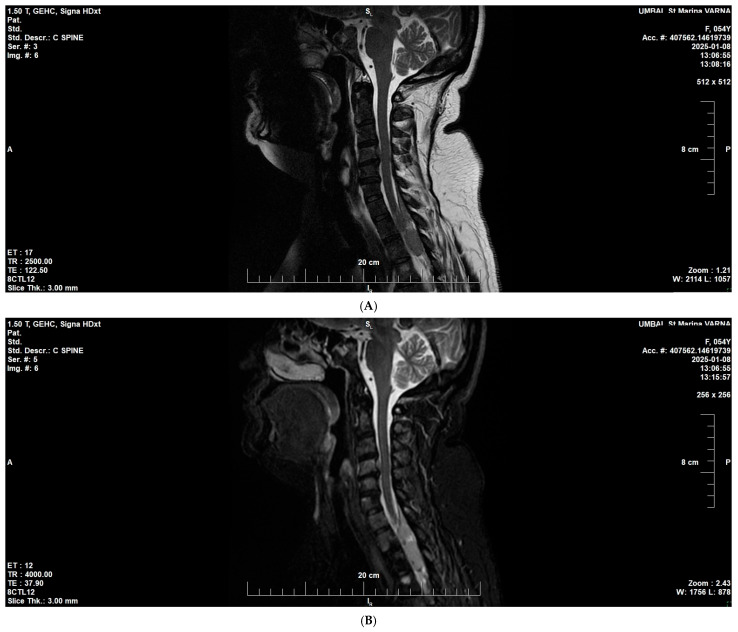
Cervicothoracic MRI at disease progression (January 2025). (A) Sagittal T2-weighted image showing diffuse bone marrow infiltration consistent with multiple myeloma. (B) Sagittal T2/STIR image demonstrating epidural soft-tissue extension at C4–C5 and C7–T2, causing thecal sac compression at T2–T3, without radiological evidence of myelopathy.
